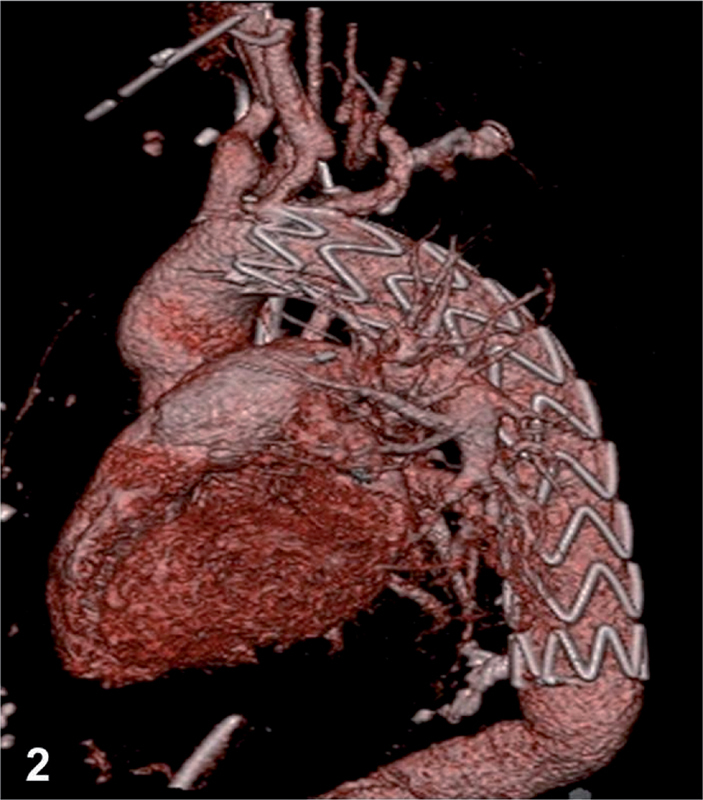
Three-dimensional reconstructed CT scan after thoracic endovascular stent graft implantation.
